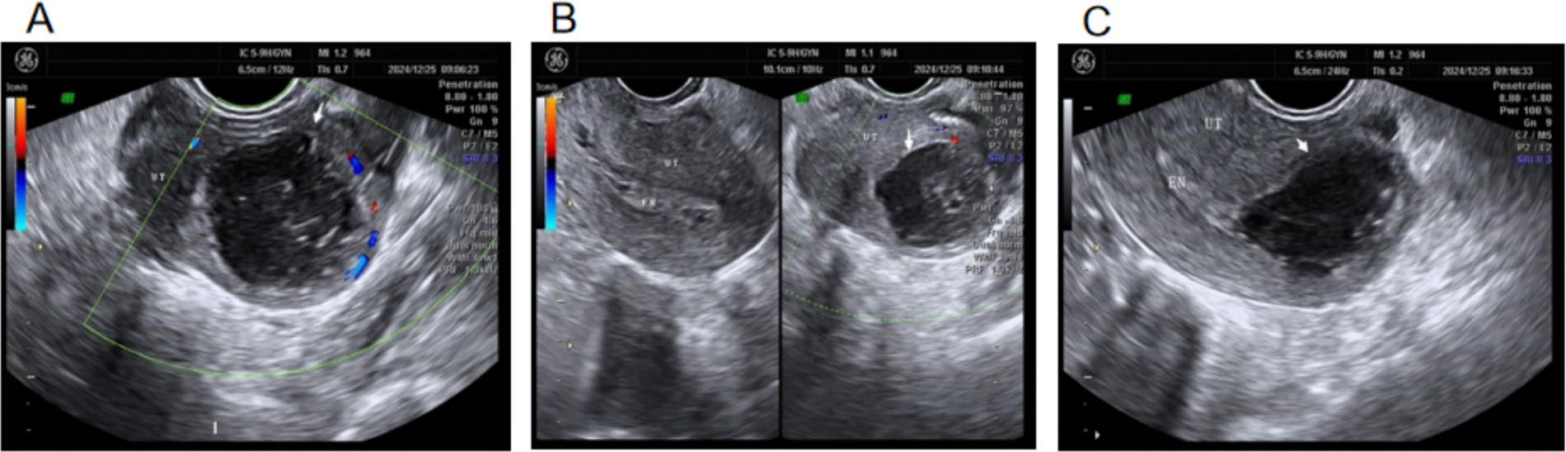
Gynecologic ultrasound images on 5 days after discharge. (A–C) White arrows: necrosis and liquefaction of the left interstitial tubal pregnancy connected to the uterine cavity.
